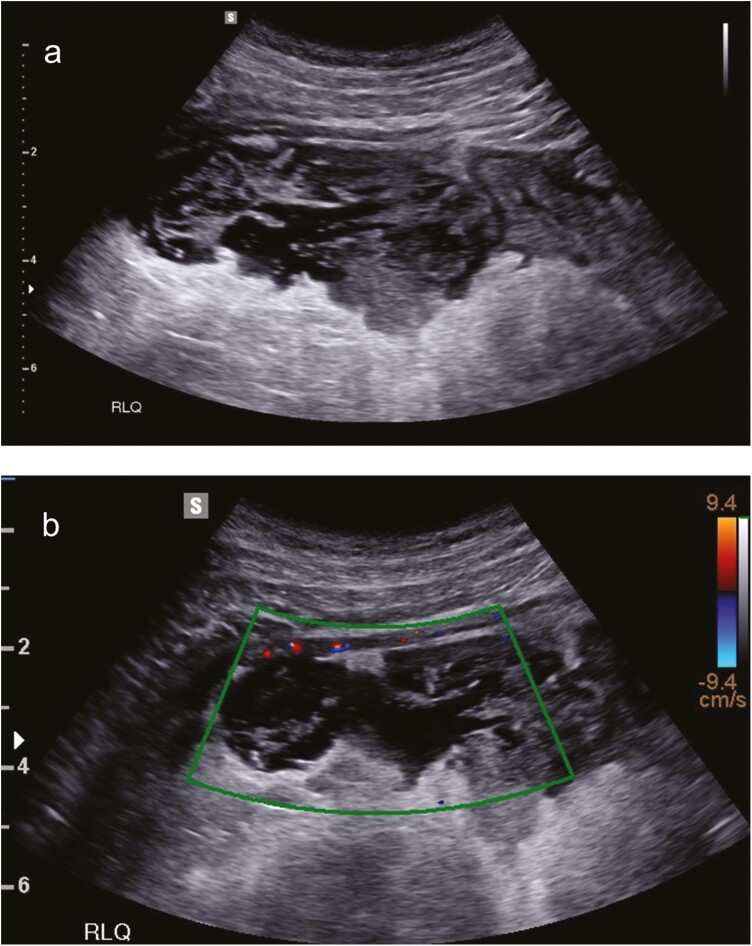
Patient with a postoperative anastomotic leak and abscess. A patient with Crohn’s disease (CD) and 9 days post-ileocecectomy was assessed at the inflammatory bowel disease (IBD) flare clinic for abdominal pain and leukocytosis. (A) A 8-cm abscess at the site of the anastomosis was identified, with surrounding hyperechoic inflammatory fat. (B) The abscess showed a lack of blood flow, as demonstrated by lack of Doppler signal, and consistent with pus. Images were acquired with a multifrequency convex transducer (3–10 MHz) with a Samsung RS80A machine (Samsung Medison Co. Ltd, Seoul, Korea).
